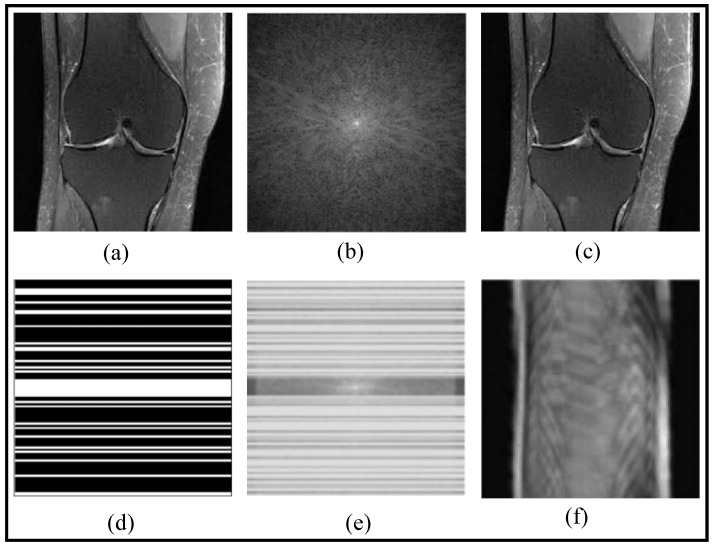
Fast MRI Knee Image Reconstruction: From Fully Sampled to Aliased Images: (a) Reconstructed MRI obtained from DL method (*X*), (b) $y^{\mathrm{Full}}$, (c) $X^{\mathrm{Full}}$, (d) *M*, (e) *y*, and (f) $X^{\mathrm{aliased}}$ (adapted with changes from [5]).
